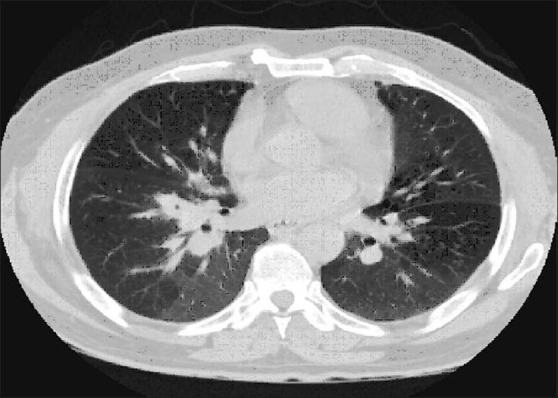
Patient 4. HRCT scan at the mid lung zones showing conglomeration pattern with background diffuse hyperinflation. Clinical characteristics of the patient are shown in Table 4
